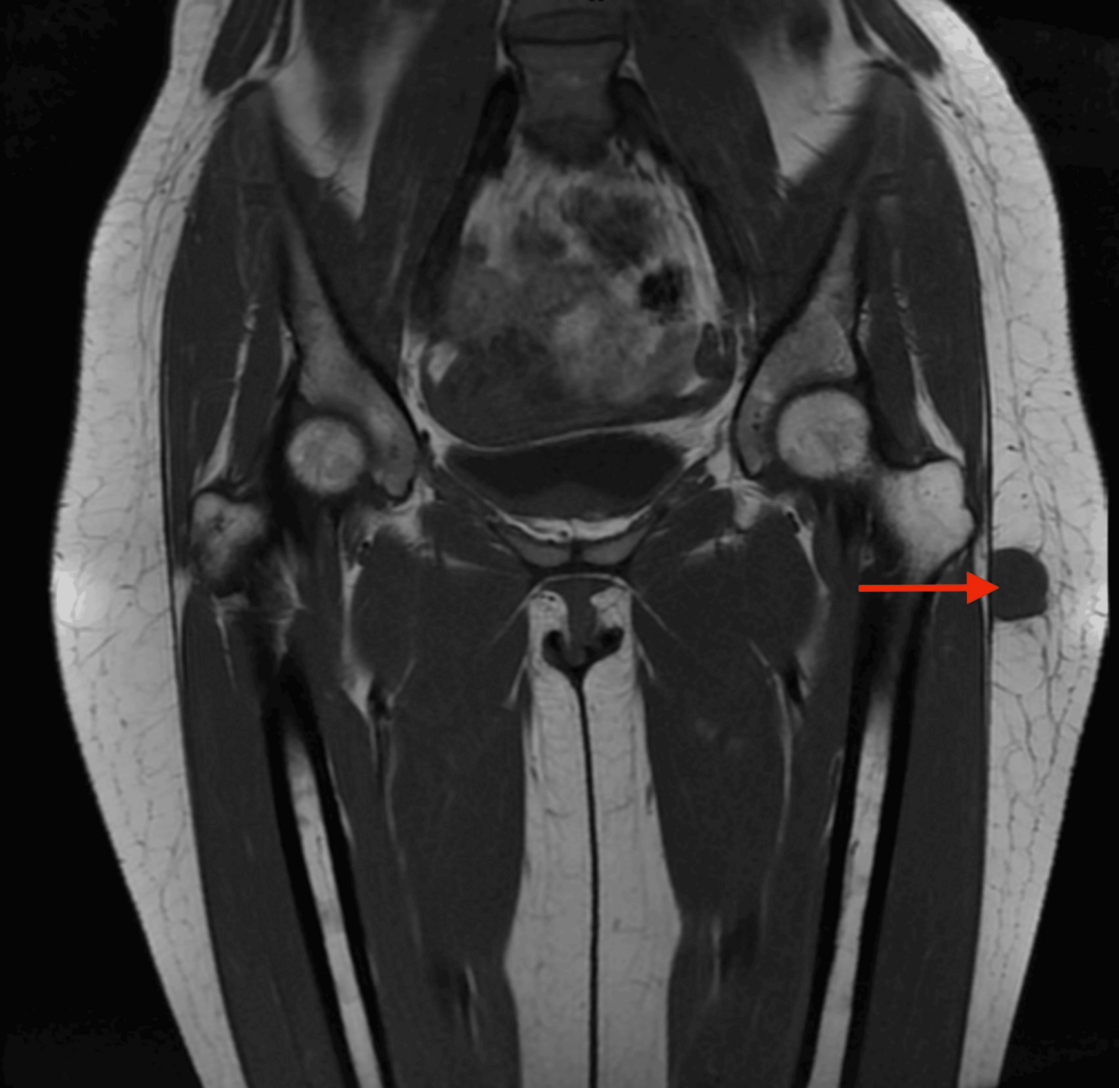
Coronal T1-weighted MRI of the pelvis shows a well-circumscribed nodular lesion in the superficial soft tissues along the lateral aspect of the left hip, adjacent to the tensor fasciae latae (red arrow). The lesion is isointense to skeletal muscle, with no adjacent bone involvement.
